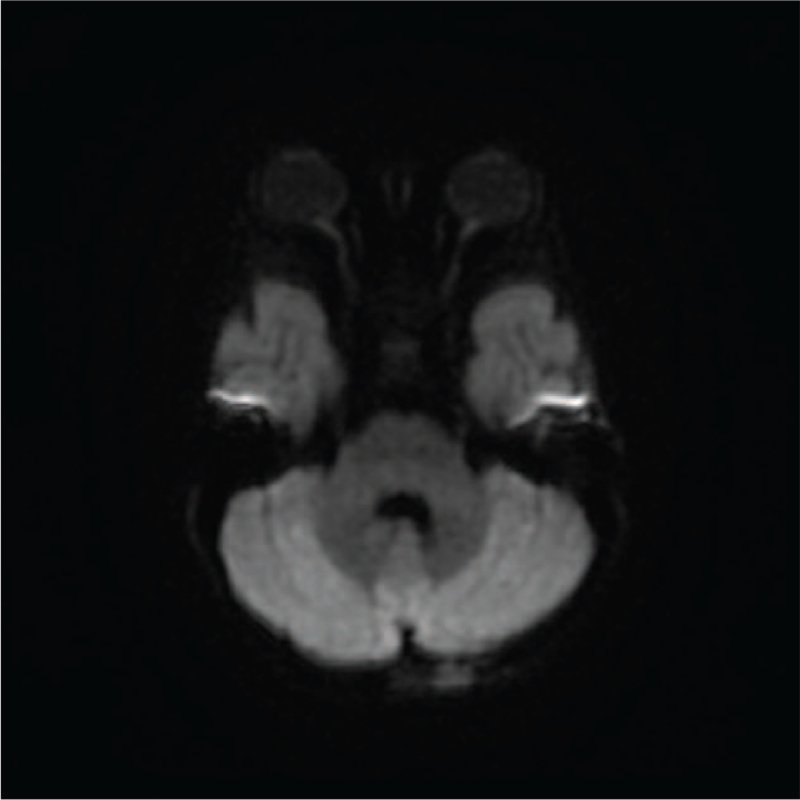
Brain magnetic resonance imaging (MRI) demonstrated complete remission of lesions in the bilateral middle cerebellar peduncles on diffusion-weighted imaging (DWI).
